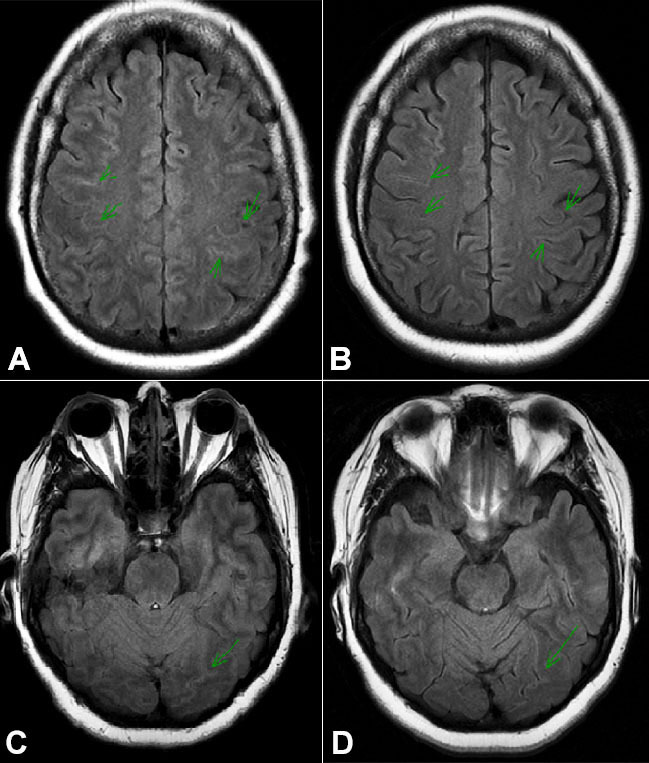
Axial T2 FLAIR MRI sequences of the brain show a diffuse subtle hyper-intense signal of the sulci when done two weeks after the patient received Atezolizumab (A and C), suggestive of the leptomeningeal irritation (could be related to leptomeningeal spread of disease, meningitis, or an inflammatory process), and interval resolution of the sulcal hyper-intense signal after a few months (B and D). There was no abnormal intra-parenchymal signal on FLAIR.
